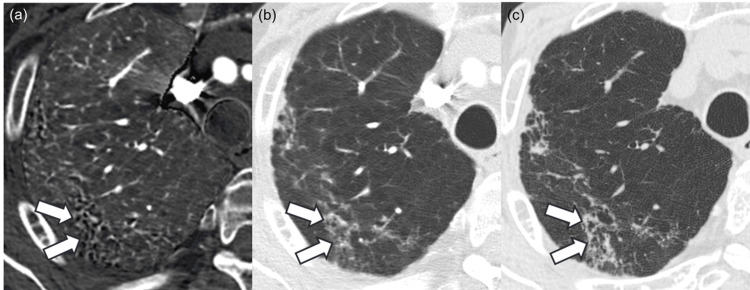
Images of a patient diagnosed with rheumatoid arthritis. The iodine map (a) and monochromatic image (b) of a 79-year-old male patient diagnosed with rheumatoid arthritis. Bronchiectasis and airspace enlargement, which are presented as black tiny structures in the region surrounded by white arrows, are more prominent in the iodine map compared to the monochromatic image, and readers 1, 2, and 3 rated 2 (iodine map is strongly prominent), 1 (iodine map is prominent), and 2 in side-by-side qualitative evaluation, respectively. Bronchiectasis and airspace enlargement progressed in the evaluated region in the follow-up CT examination (c), which was performed after 127 days. Window level/width for iodine map (a) and CT images (b, c) were 15/110 mgI/ml and –600/1600 Hounsfield unit, respectively.
